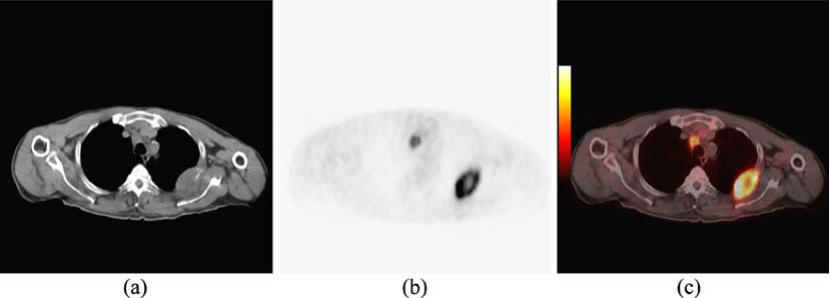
a CT, b PET, and c PET/CT fused image of a patient having EPTB.
